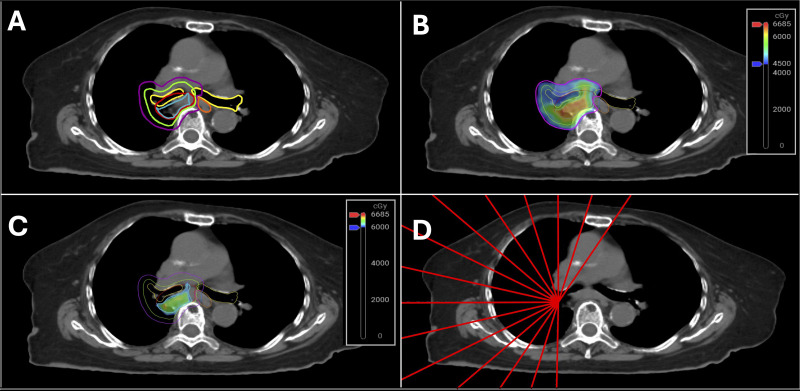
CT axial view with pre-set soft tissue window/level. (A) Demonstrated are the critical OARs–the PBT (yellow) and Esophagus (orange). Additionally, the IGTV_4500 (red), ITV_4500 (green) and the PTV_4500 (purple). The IGTV_6000 (cyan) is derived from the PTV_4500 minus the OAR_PRV (3mm expansions of OARs). (B) demonstrates the 4500cGy prescription dose, (C) demonstrates the dose escalation volume, and (D) demonstrates an example beam geometry.
